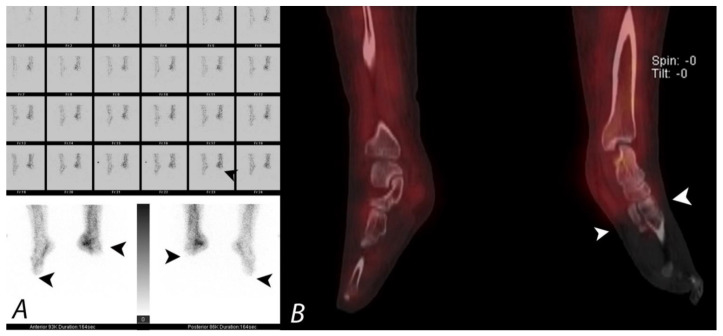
Patient presenting with severe cold injury (frostbite) to bilateral feet underwent three phase bone to evaluate for the extent of devascularization as a part of presurgical evaluation. Blood flow images and blood pool images (A) demonstrate absent perfusion and pool activity involving the fore foot and mid foot on the left side and involving the forefoot on the right side. Coronal SPECT/CT (B) confirms the findings with accurate delineation and demarcation of viable and non-viable tissue. Patient underwent bilateral below knee amputation.
